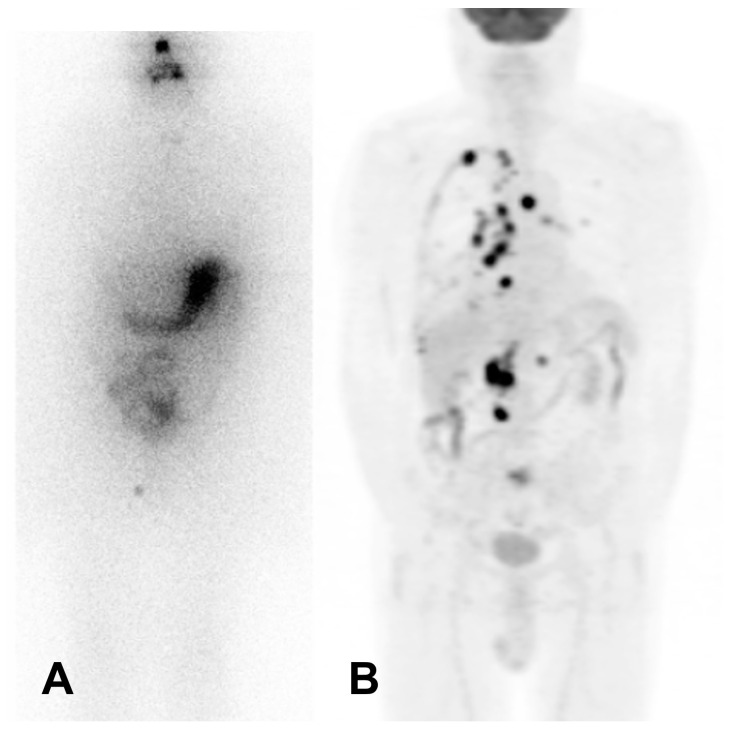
Patient with negative post-treatment whole-body radioiodine scintigraphy (A) and multiple [^18^F]FDG-avid lesions (B). Legend: male, 67 years, affected by papillary thyroid carcinoma with increasing thyroglobulin level 2 years after thyroidectomy and radioiodine therapy [4.4 GBq]. (A) Post-treatment whole-body scintigraphy [7.4 GBq] showing no radioiodine-avid lesions. (B) [^18^F]FDG PET/CT: multiple [^18^F]FDG-avid lesions in the mediastinum and lung, with an associated [^18^F]FDG-active pleural effusion (right side), and [^18^F]FDG-avid lesions in the retroperitoneal region. A biopsy on an abdominal lesion confirmed metastasis of papillary thyroid carcinoma.
